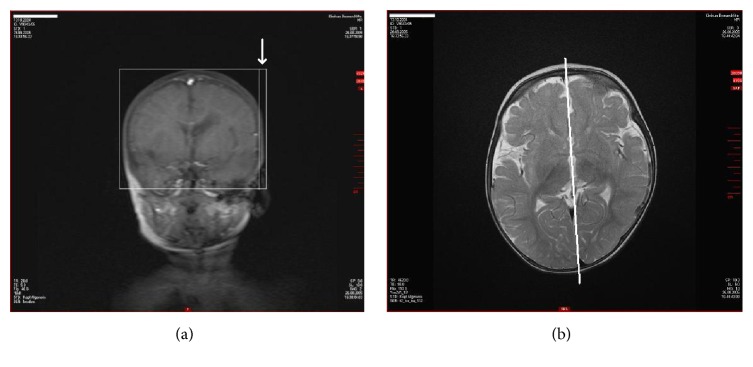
MRI of the head (M.H.) at 10 months of age (2005). (a) Significant depression of parietal bone left in a.p. projection (molding, arrow) (1.5-Tesla Avanto, head coil array, T1w TSE sequence). (b) Obvious volume reduction of the left hemisphere by birth trauma with ischemic stroke (1.5-Tesla Avanto, head coil array, T2w TSE sequence).
